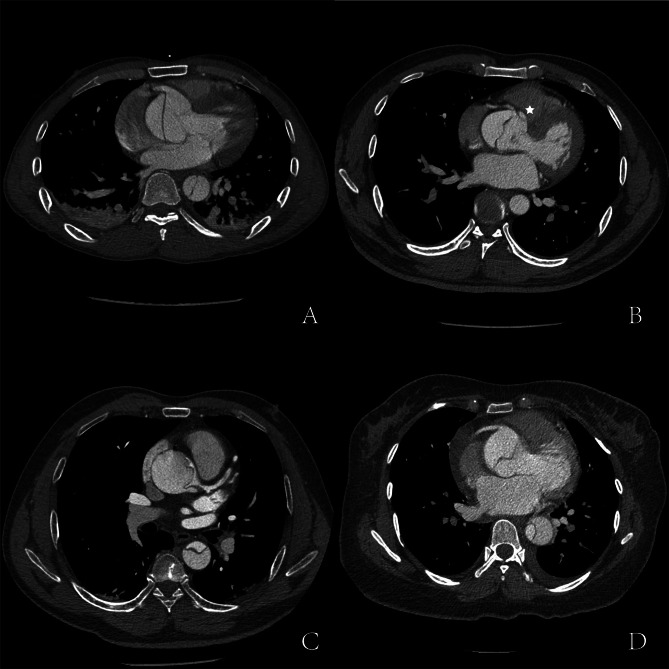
Manifestations of coronary artery involvement on computed tomography angiography A, type S1, dissection involving half of the ostium (right coronary) B, type S1, dissection involving a circle of the ostium; asterisk, false lumen under the ostium(right coronary) C, type S2, dissection extending to coronary trunk with thrombosis in false lumen(left coronary) D, type C2, coronary trunk originated from false lumen(right coronary)
